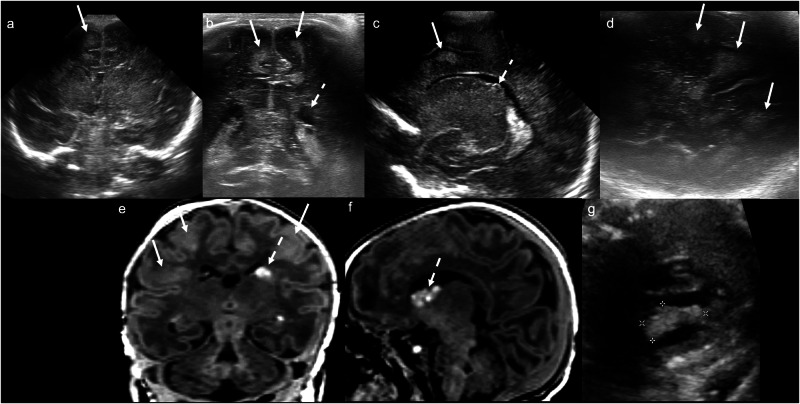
Tuberous sclerosis. Day 1 ultrasound in a 32-week gestation neonate with a paternal history of tuberous sclerosis and an antenatally detected cardiac rhabdomyoma. a, b Coronal and (c, d) parasagittal images from day 1 (postnatal) ultrasound demonstrate multiple confluent areas of increased echogenicity in the cortical/subcortical and deep white matter of both cerebral hemispheres (solid arrows), without posterior acoustic shadowing; subependymal nodules are also evident (dashed arrows). e Coronal and (f) parasagittal T1WI shows the corticosubcortical tubers as subtle, ill-defined T1 hyperintense, radially oriented lesions (solid arrows); the T1 hyperintense subependymal nodules (dashed arrows) are more conspicuous than on CUS. g Antenatal ultrasound demonstrated an echogenic intracardiac lesion (measured), consistent with a rhabdomyoma
